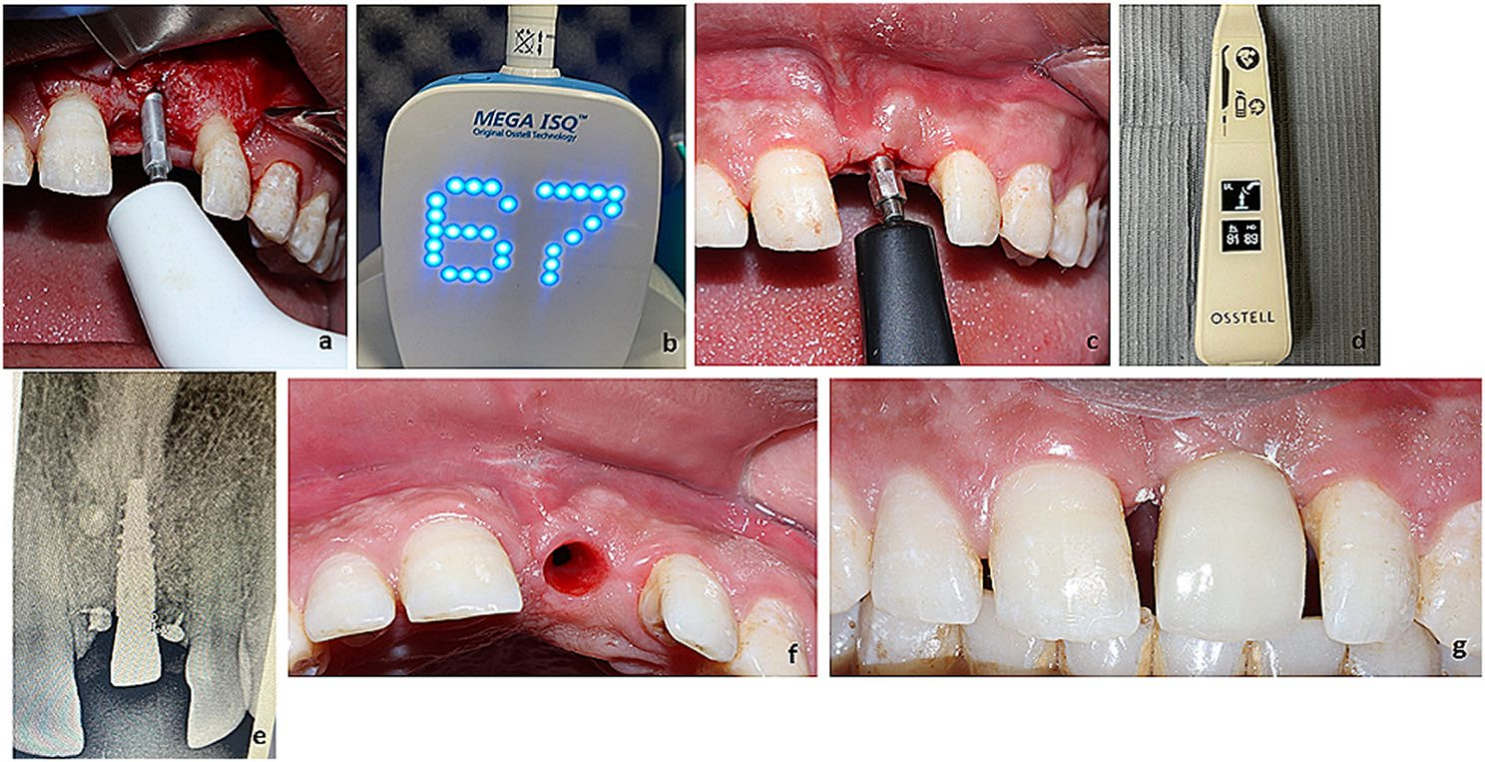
a, b. Primary implant stability measured after insertion (6 months from augmentation) that was 67 ISQ, c, d: secondary implant stability (6 months from implant placement) that was 89 ISQ, e: periapical radiograph of implant, f: implant uncovering showing a healthy pink gingival cuff, g: frontal view of final restoration of tooth immediately after cementation
